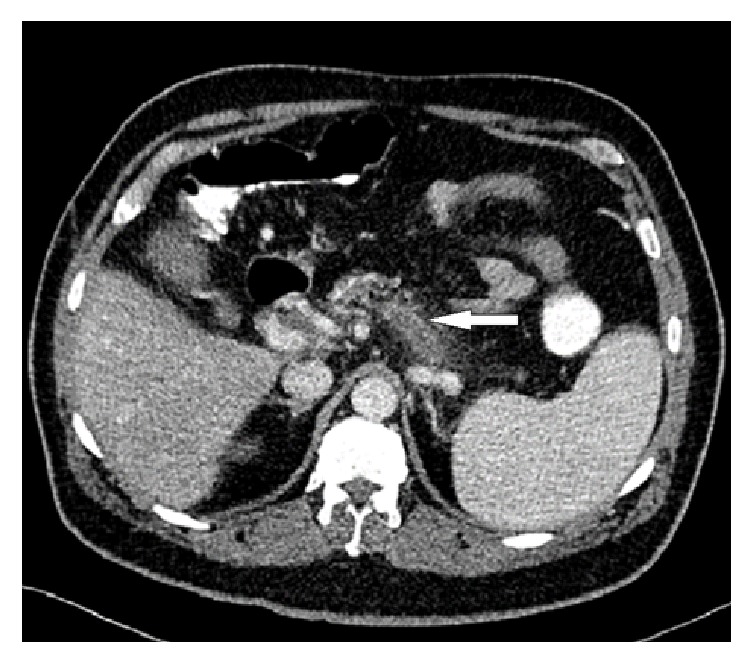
Abdomen CT scan with contrast (axial image) shows distended splenic vein with filling defect, indicating thrombosis (white arrow).
